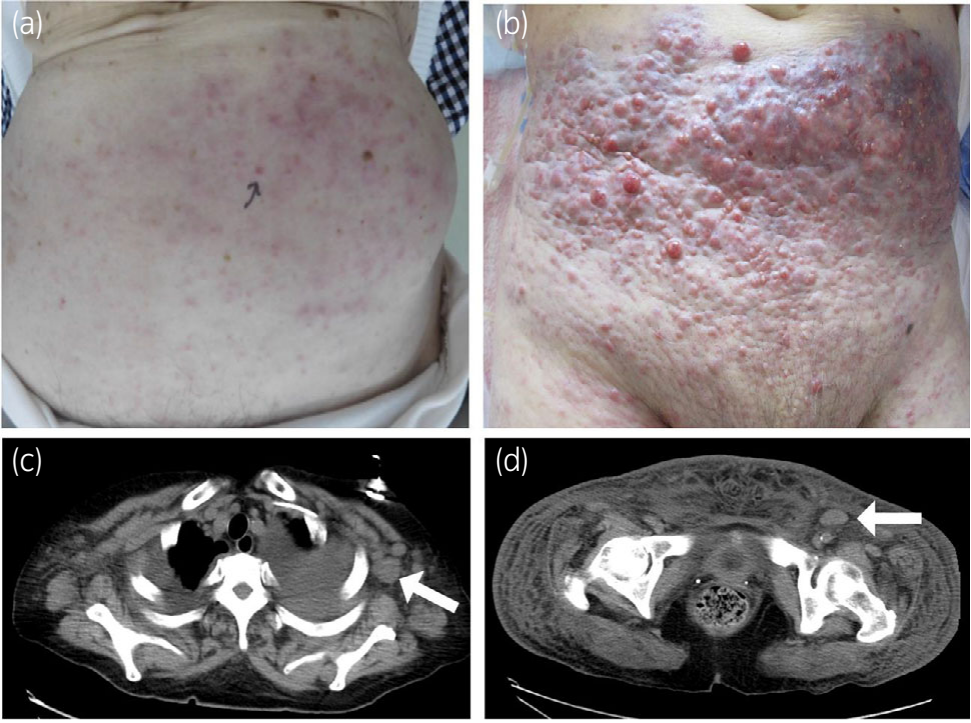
(a) Skin lesions on the lower abdominal wall before pembrolizumab therapy harboring multiple nodules with redness. (b) Skin lesions on the lower abdominal wall after pembrolizumab therapy. Infiltrative erythema and nodules with fusion. (c) Left axillary lymph node swelling on the computed tomography (CT) before pembrolizumab therapy (arrow). (d) Left inguinal lymph node swelling and subcutaneous infiltration on CT before pembrolizumab therapy (arrow).
